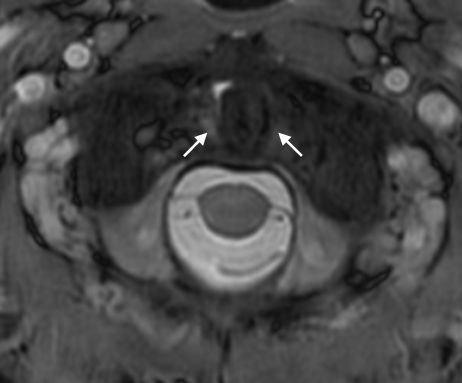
Atlantoaxial magnetic resonance imaging. Arrows point to the intact alar ligaments.
